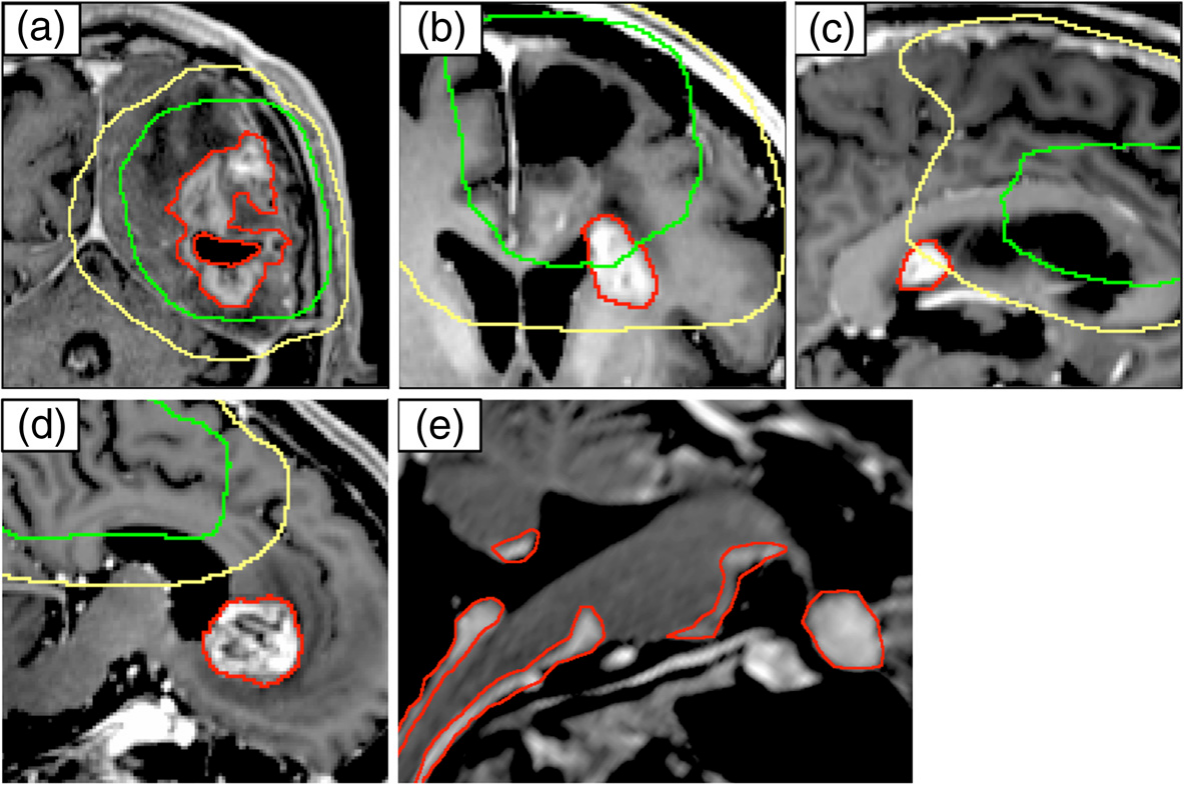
Examples of recurrence patterns. Examples of recurrence patterns on T1-weighted magnetic resonance imaging with contrast: central (a), in-field (b), marginal (c), out-field (d), and distant recurrences (e). Red contours indicate recurrent tumors. Green and yellow lines indicate 95% isodose lines of 60 Gy and 50 Gy, respectively.
